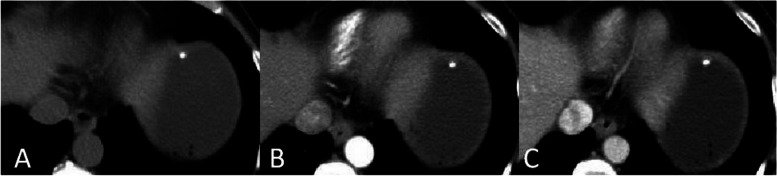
With a single submucosal stromal tumor. A Unenhanced CT scan showed nodular calcifications under the mucosa of the gastric fundus. CT scan in the arterial phase (B) and portal vein phase (C) showed the calcifications partially protruded into the gastric cavity, calcifications were surrounded by a few solid components, and no heterogeneous enhancement around the calcification foci
